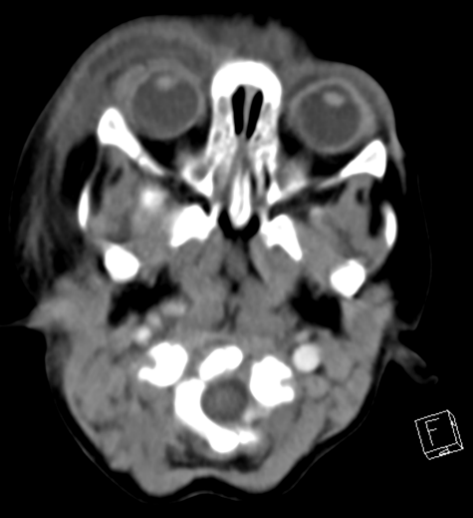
Computed tomography (axial scan) of the orbit showing extensive swelling of the right periorbital soft tissues and minimal swelling on the left side, without intraorbital involvement
